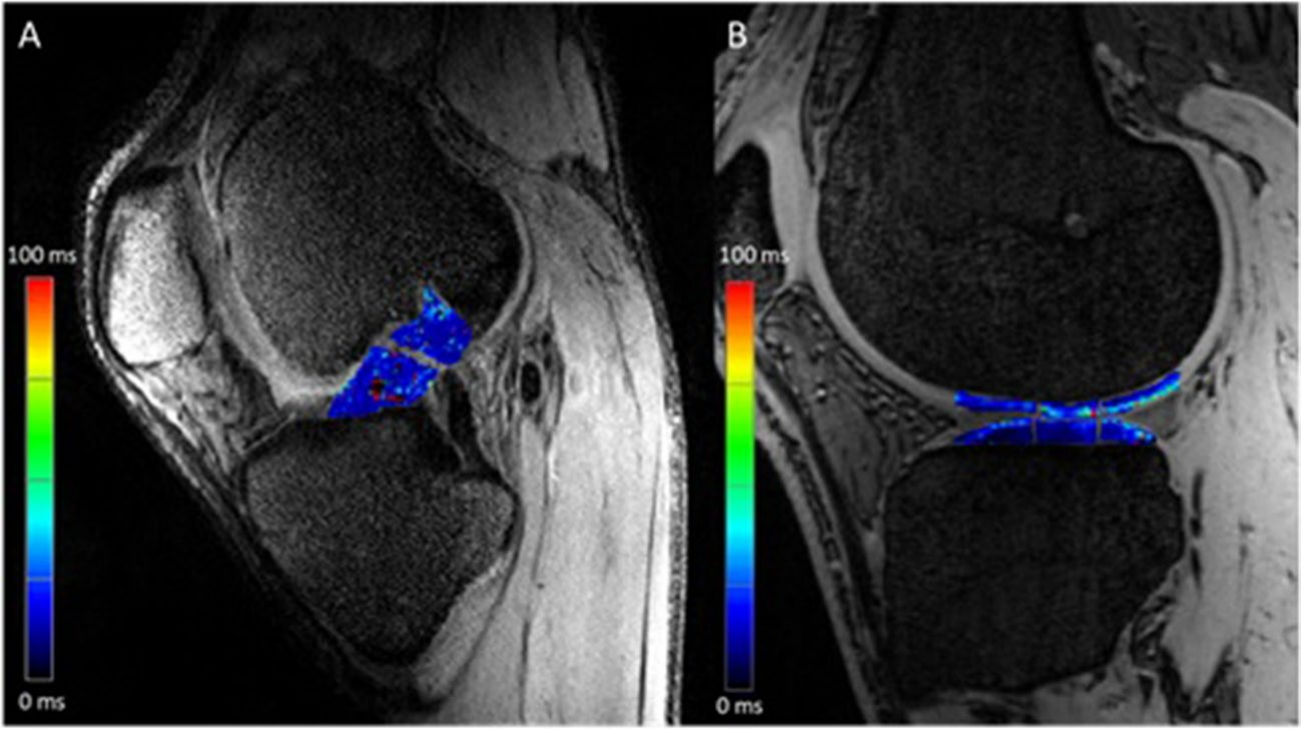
A 7T MRI image of the first echo of parasagittal T2 mapping with merged regions of interest of the corresponding T2 map for the anterior cruciate ligament of a 26-year-old female patient after ACL repair. B Image of the first echo of sagittal T2* mapping with merged regions of interest of the corresponding T2* map for the tibial and femoral cartilage
